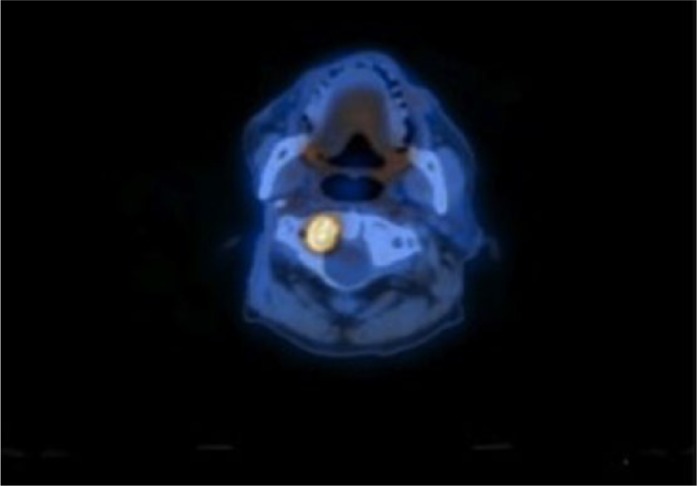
PET/CT scan shows the recurrence of the carcinosarcoma including a metastatic lesion that is in the C1 region.
